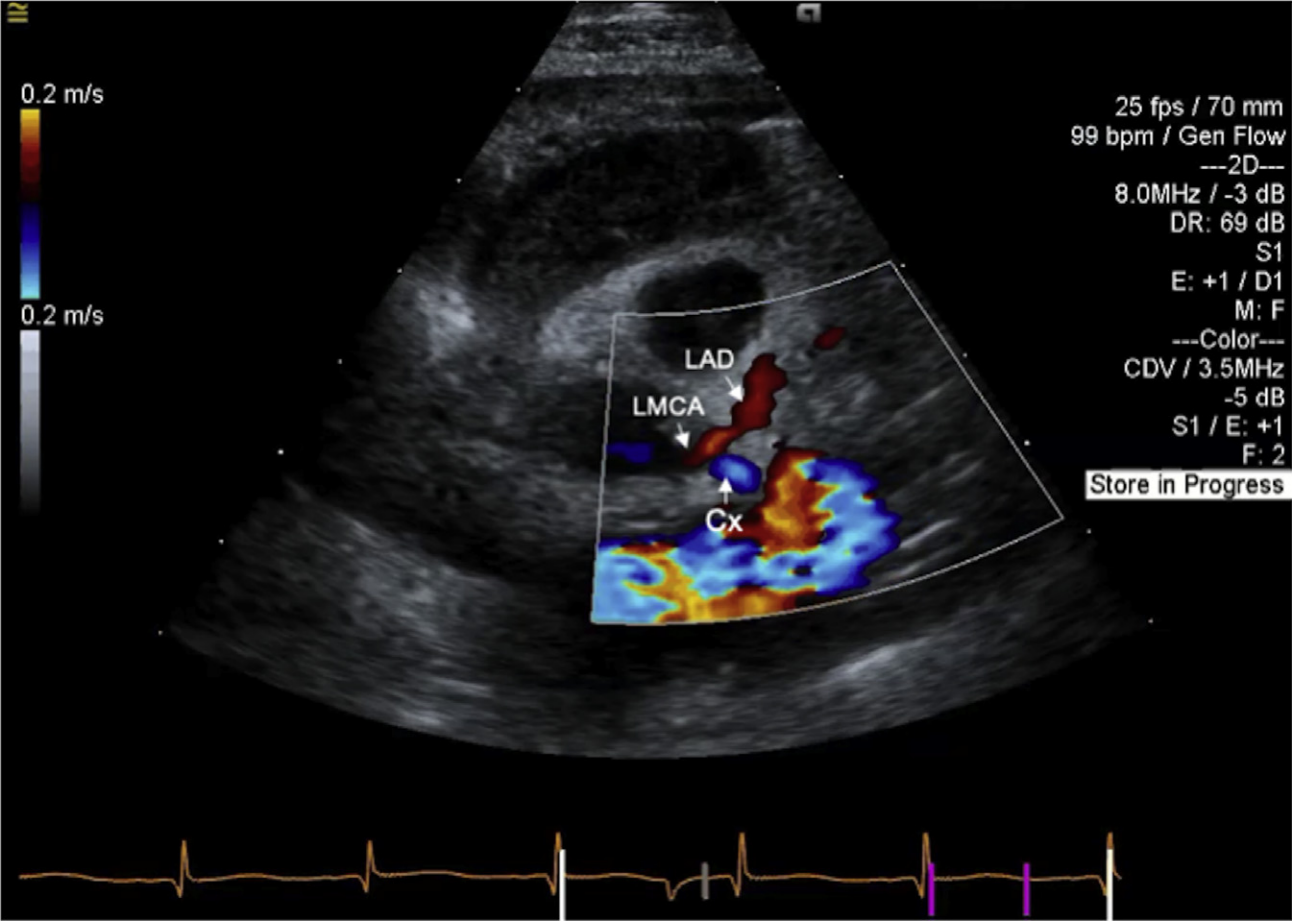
Postoperative transthoracic echocardiogram demonstrates normal antegrade filling of the left coronary artery following opening of the left coronary ostia with homograft patch reconstruction of the proximal coronary artery. *Cx*, Circumflex artery.
